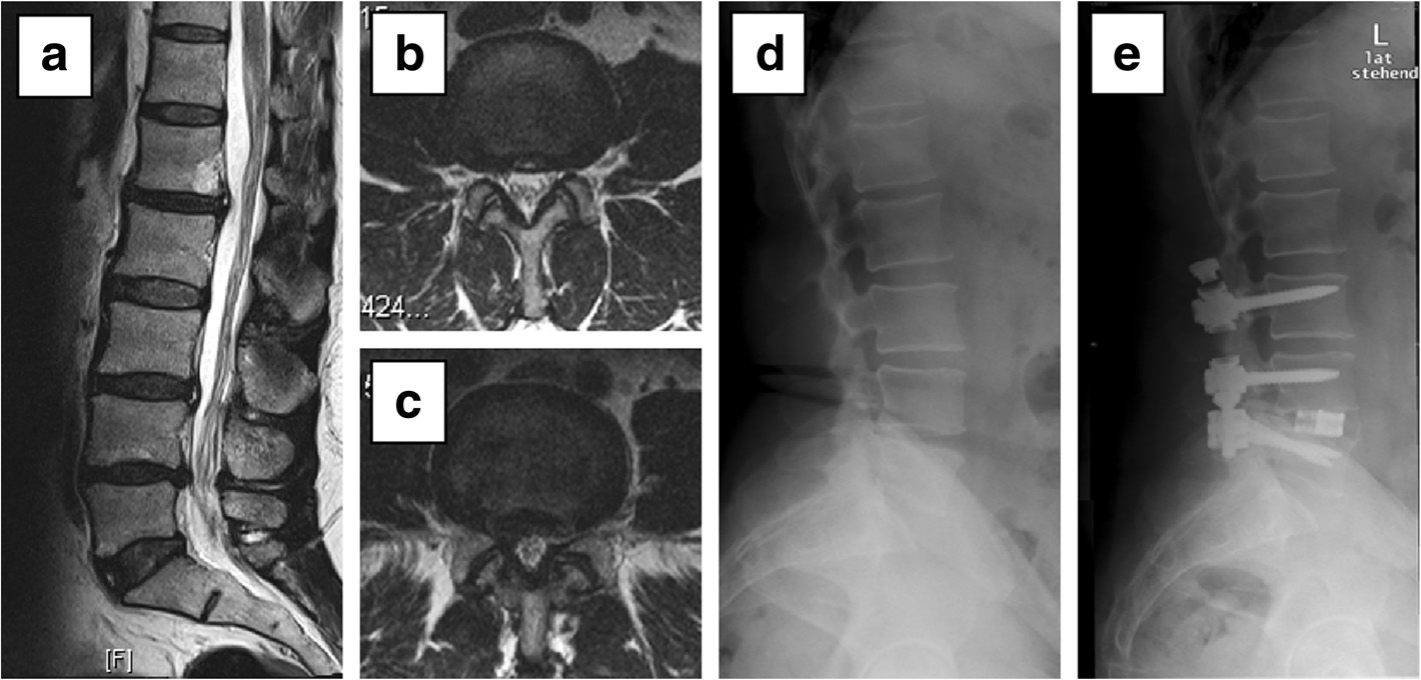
Semirigid inclusion of the cranial motion segment after IGD. a-c T2-weighted magnetic resonance images preoperatively: a lumbar sagittal image and b axial lumbar image of L3/4 and c L4/5. d Lateral radiographic image preoperatively and e postoperatively. The patient presented with chronic lumbar back, strong sciatic pain bilaterally, and a hypoesthesia at the right lateral lower leg. Moderate intervertebral disc degeneration was observed at L3/4 and L5/S1 (Pfirrmann grade 3), advanced degeneration at L4/5 (Pfirrmann grade 4-5), and a neuroforaminal stenosis on the right side due to facet joint hypertrophy and intervertebral disc protrusion (grade 2 according to Lee [16, 17]). Moderate facet joint degeneration was seen at L3/4 and L4/5 (Weishaupt grade 1). Recommendation before inpatient gradual diagnostics (IGD): lumbar fusion of L4/5 with transforaminal lumbar interbody fusion (TLIF). Results obtained during IGD: • bilateral facet joint infiltration of L3/4: 30 % pain relief back pain. • bilateral facet joint infiltration of L4/5: 30 % pain relief back pain. • epidural injection of L4/5: 30 % sciatic pain relief. • bilateral sacroiliac joint infiltration: 0 % pain relief. Although the absolute values for pain relief were only at moderate levels, the patient’s walking distance increased dramatically after the infiltrations. Recommendation after IGD: lumbar fusion of L3-5 with TLIF L4/5 and a semirigid instrumentation of L3/4. At the 3-month follow-up, the patient reported considerable pain relief and an increase in walking distance in comparison to that achieved preoperatively
